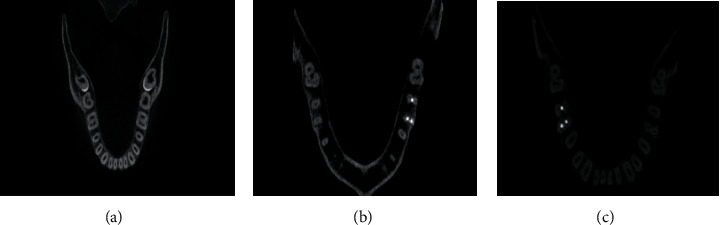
Axial CBCT sections for C-shaped canals. (a) Type C1 in tooth #47. (b) Type C2 in tooth #37. (c) Type C2 in tooth #47.
